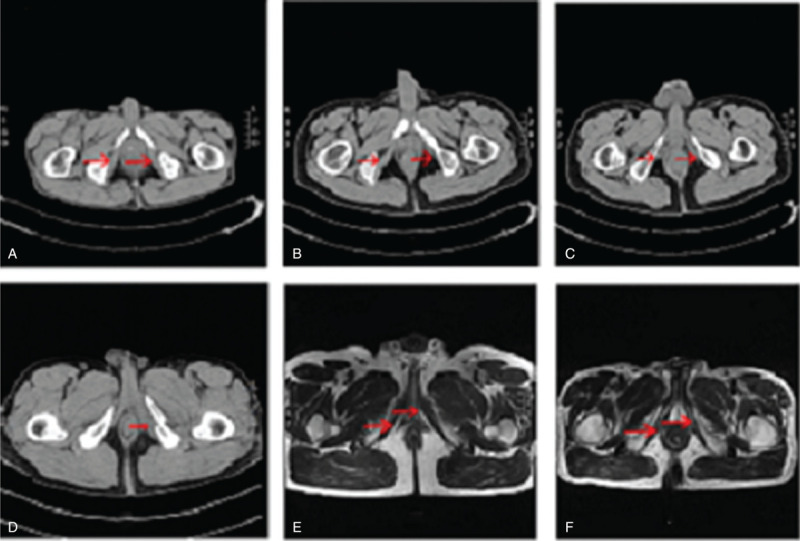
The appearance of ischiocavernosus muscle injury is shown by computed tomography/magnetic resonance imaging after pelvic fracture. (A) The normal ICM; (B, C) avulsion of the starting tendon of the ICM; (D) ICM swelling, hematoma formation, starting tendon avulsion injury, and the fracture end embedded into the ICM; (E, F) in the chronic phase, the impaired ICM demonstrated a speckle formation on T2WI showing low signal, and muscle atrophy and fat infiltration on T1WI showing high signal (→ was shown in). The area indicated by the red arrow is ICM injuries.
